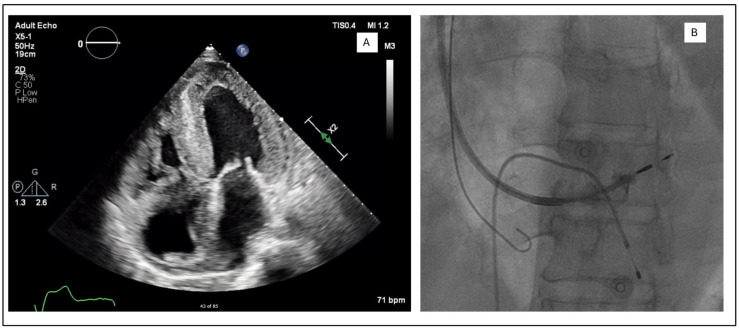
(A) Apical four chamber view showing biventricular wall thickening and biatrial dilatation. (B) Use of sheath-in-sheath technique with an extended hook to provide additional support during the deployment of the Medtronic SelectSecure 3830 lead. Venogram shows that the ring is deeply embedded in the septum.
